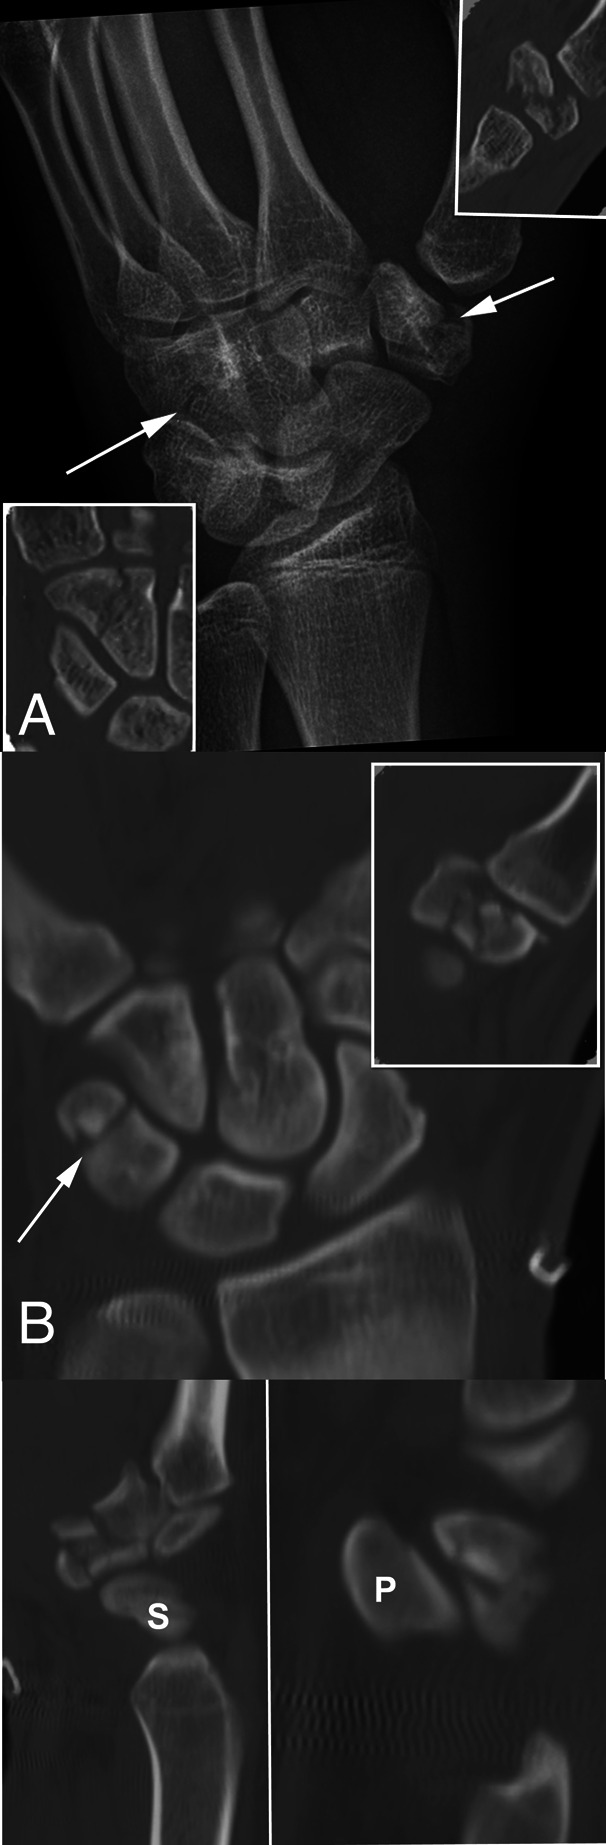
A, An 18-year-old right-hand–dominant man fell while riding a dirt bike. He was noted to have a comminuted fracture of the trapezium. CT was conducted to better evaluate his injury pattern. Findings included a comminuted fracture of the left trapezium with marked crush component and a nondisplaced hamate body fracture. CT images of the trapezium and hamate are shown superimposed over the planar radiograph. B, A 26-year-old right-hand–dominant man sustained multiple injuries from a motorcycle accident. Upper extremity injuries included bilateral open olecranon fractures, right radial head fracture, right ulnar nerve transection, and a closed right comminuted trapezium fracture with a triquetral body fracture. The trapezial body fracture was treated with open reduction and internal fixation and bone allograft augmentation. Nonsurgical care was provided for the triquetral fracture. Coronal plane CT imaging of the triquetral fracture with superimposed trapezium fracture is shown. Sagittal plane CT imaging of the trapezium (left) and triquetrum are also shown. “P” = pisiform, “S” = scaphoid
